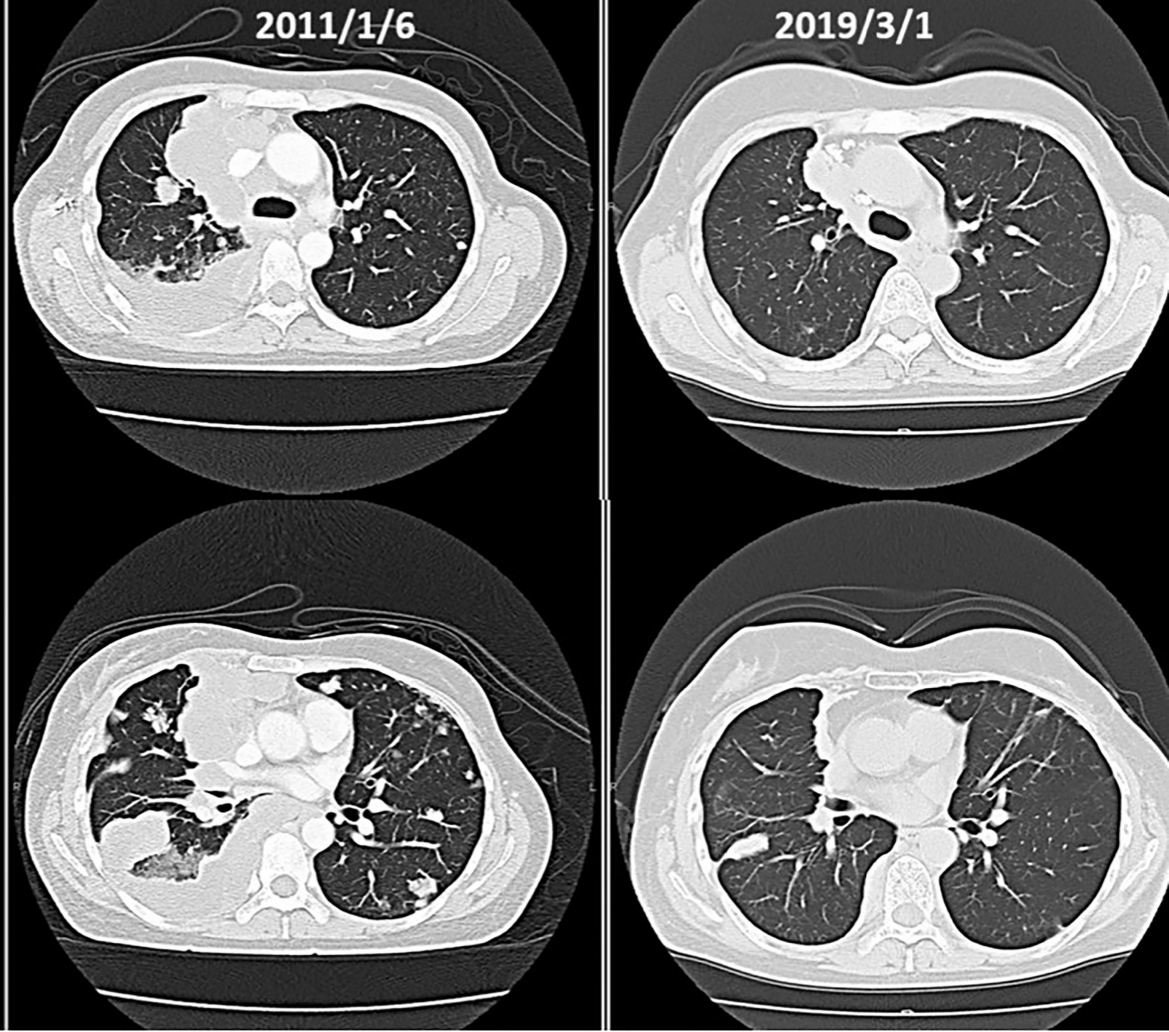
CT scans reveals gradual shrinkage of the tumors.
